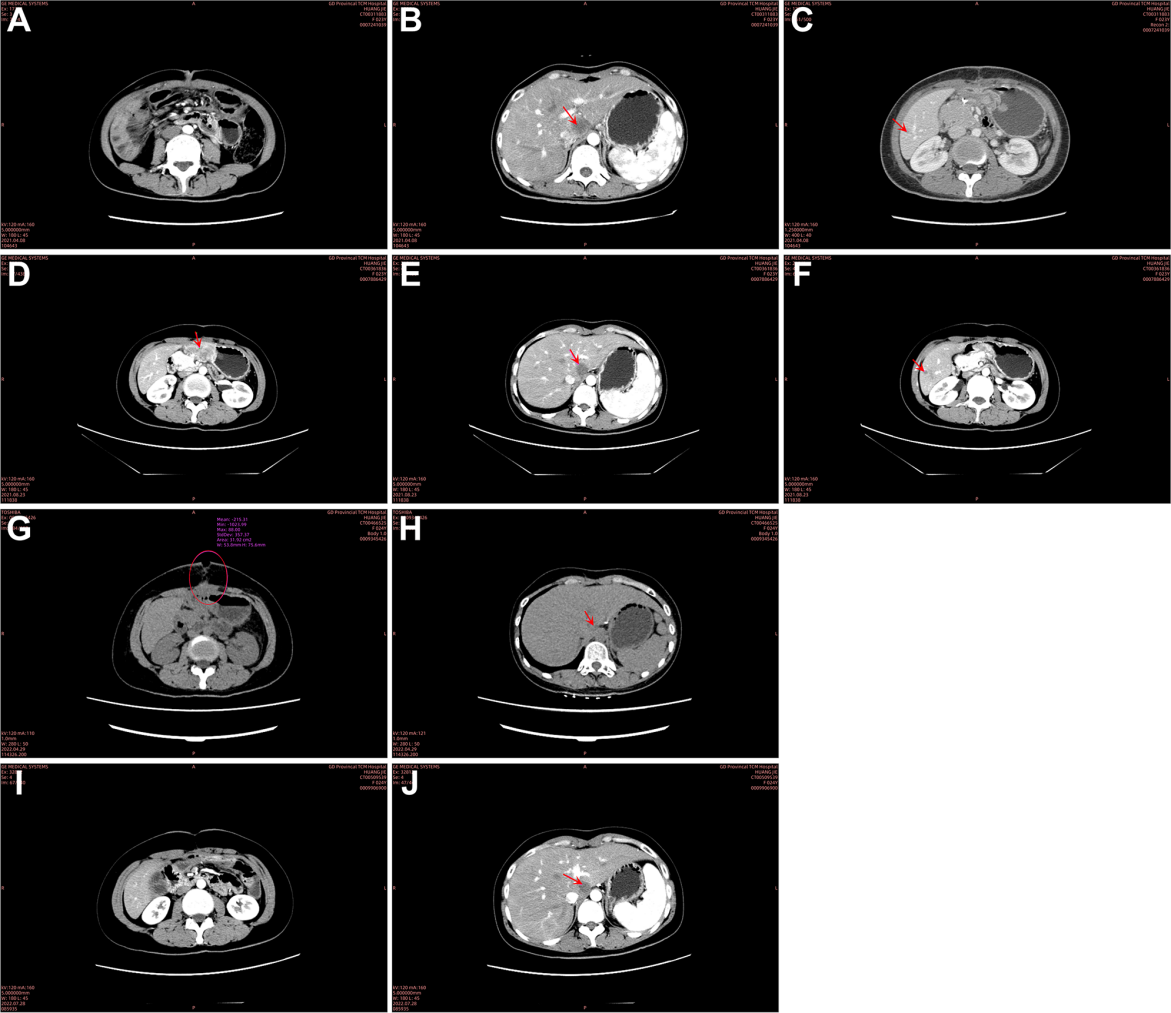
Follow-up computed tomography (CT) scans. April 8, 2021: (A) no residual mass was observed in the surgical area post-colon cancer resection. (B) The nodule at the S1–S2/3 junction of the liver (2.5 × 2.9 cm) persisted, consistent with suspected liver metastasis. (C) A new, slightly hypodense nodule (1.1 cm) was detected in the S6 segment of the liver, suspected to be a new metastatic lesion. August 23, 2021: (D) nodules and masses were identified in the gastric antrum, small omental sac, and hepatogastric space, suspicious for metastases (5.0 × 3.2 cm). (E) The nodule in the S1 segment of the liver (2.8 × 2.6 cm) remained similar to previous findings, consistent with liver metastasis. (F) The nodule in the S6 segment of the liver (1.0 cm) was also consistent with prior findings, suspected to be a liver metastasis. April 29, 2022: (G) the intestinal wall at the anastomosis site appeared unremarkable. Subcutaneous emphysema at the upper abdominal incision communicated with intra-abdominal air, forming an air-fluid level. Surrounding soft tissues were disorganized and poorly demarcated, raising suspicion for encapsulated effusion with infection and a potential entero-cutaneous fistula. (H) The nodule in the S1 segment of the liver (1.9 × 2.0 cm) persisted, consistent with a metastatic lesion. July 28, 2022: (I) the intestinal wall at the anastomosis site remained unchanged, and the subcutaneous emphysema at the original incision site had mostly resolved. Surrounding soft tissue swelling had decreased, and the anatomical structures were clearer. (J) The nodule in the S1 segment of the liver (1.9 × 2.0 cm) showed no significant changes compared to previous scans.
